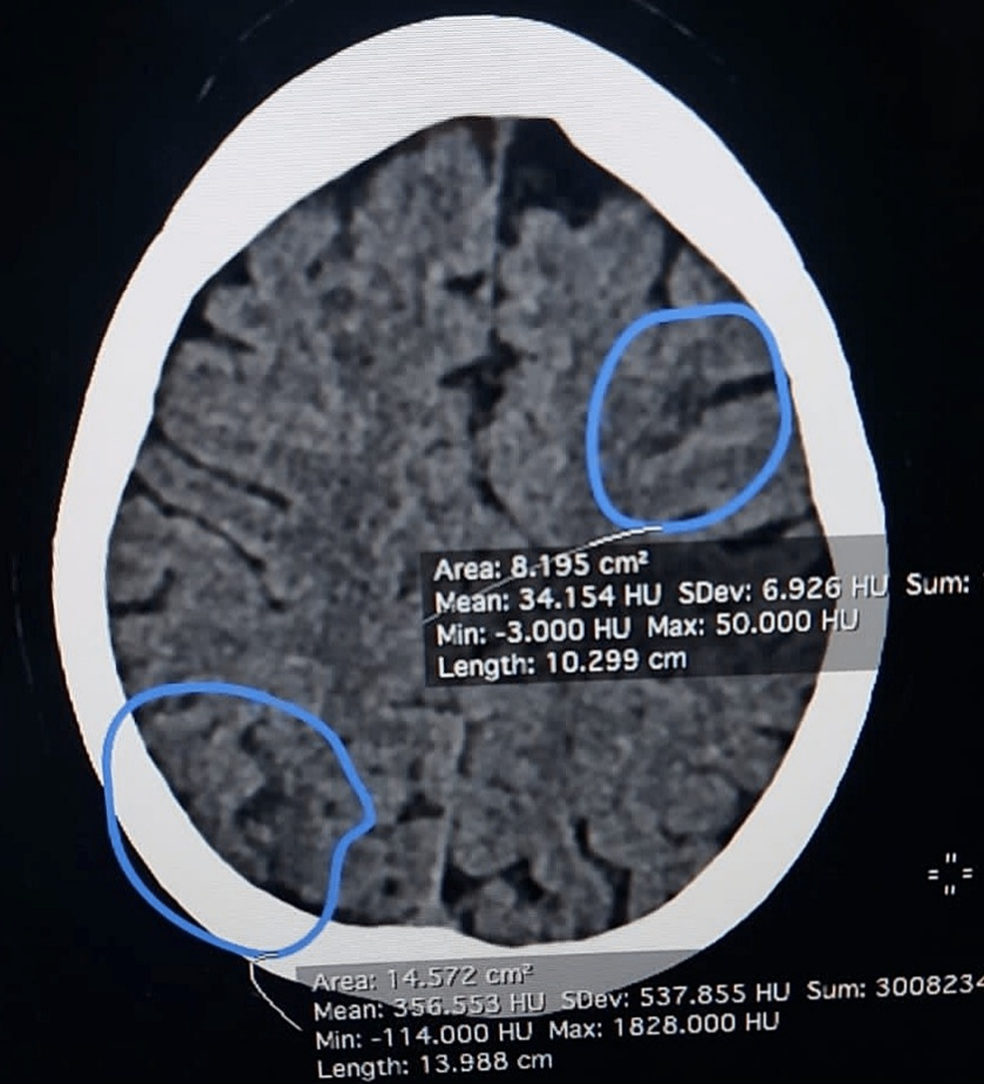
A CT brain image shows multiple acute infarcts in the right posterior parietal sulcus, right parietal subcortical white matter, left supraventricular level, and cerebellar hemisphere.
